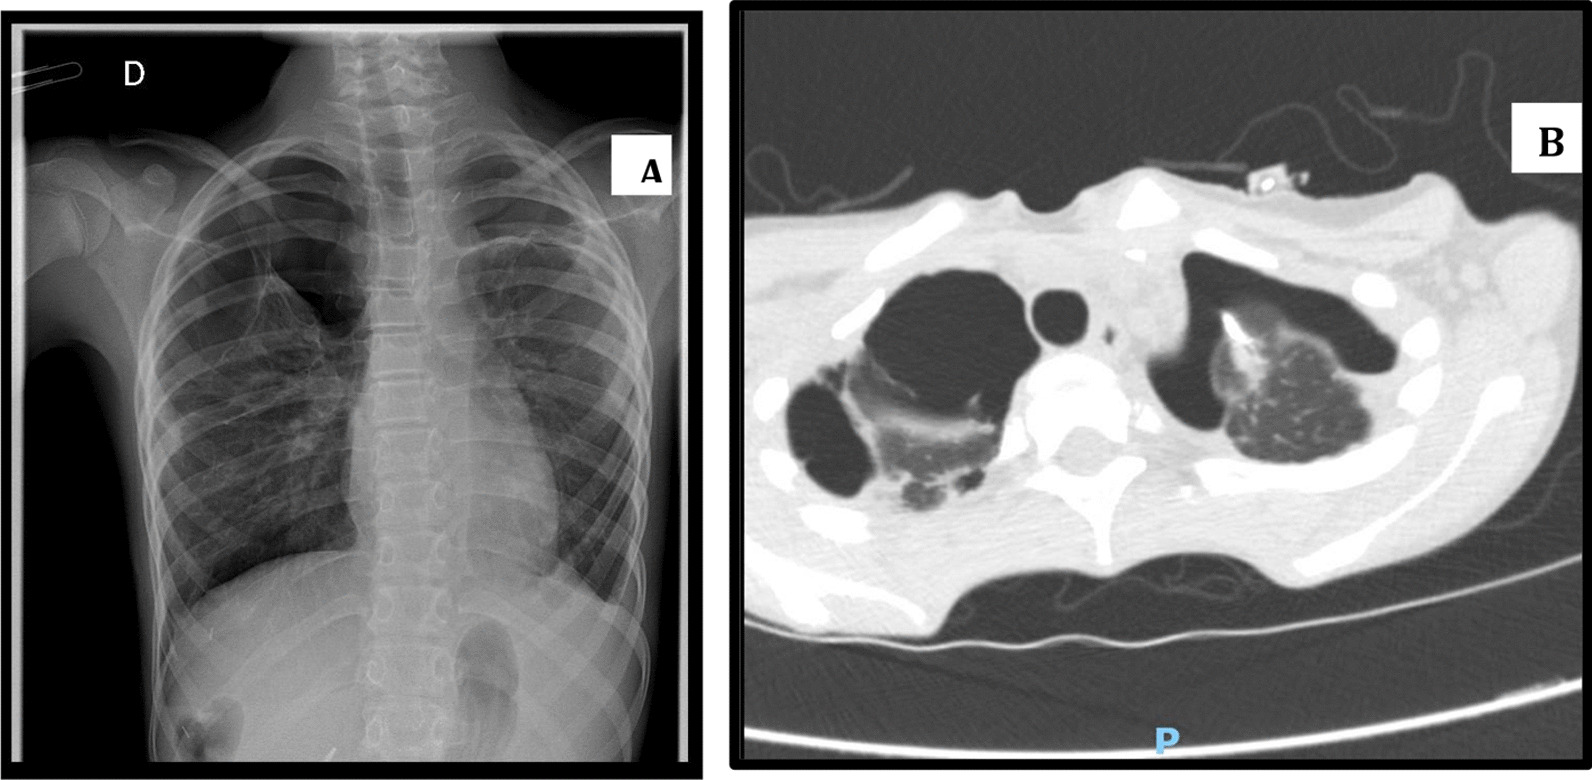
Bilateral pneumothorax in medical imaging. A Frontal chest X-ray of the patient showing a bilateral apical pneumothorax. B Thoracic computed tomography (CT) scan of the patient. Presence of a bilateral (hydro)pneumothorax with pleural thickening and predominant adhesions on the right
